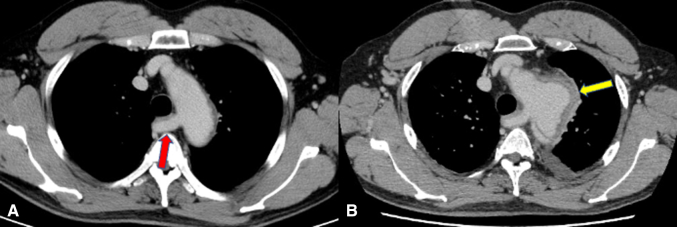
A, Computed tomography scan 3 weeks before presentation revealed an aberrant right subclavian artery (*red arrow*). B, Rapid expansion of the aneurysm to 58 mm (*yellow arrow*).
